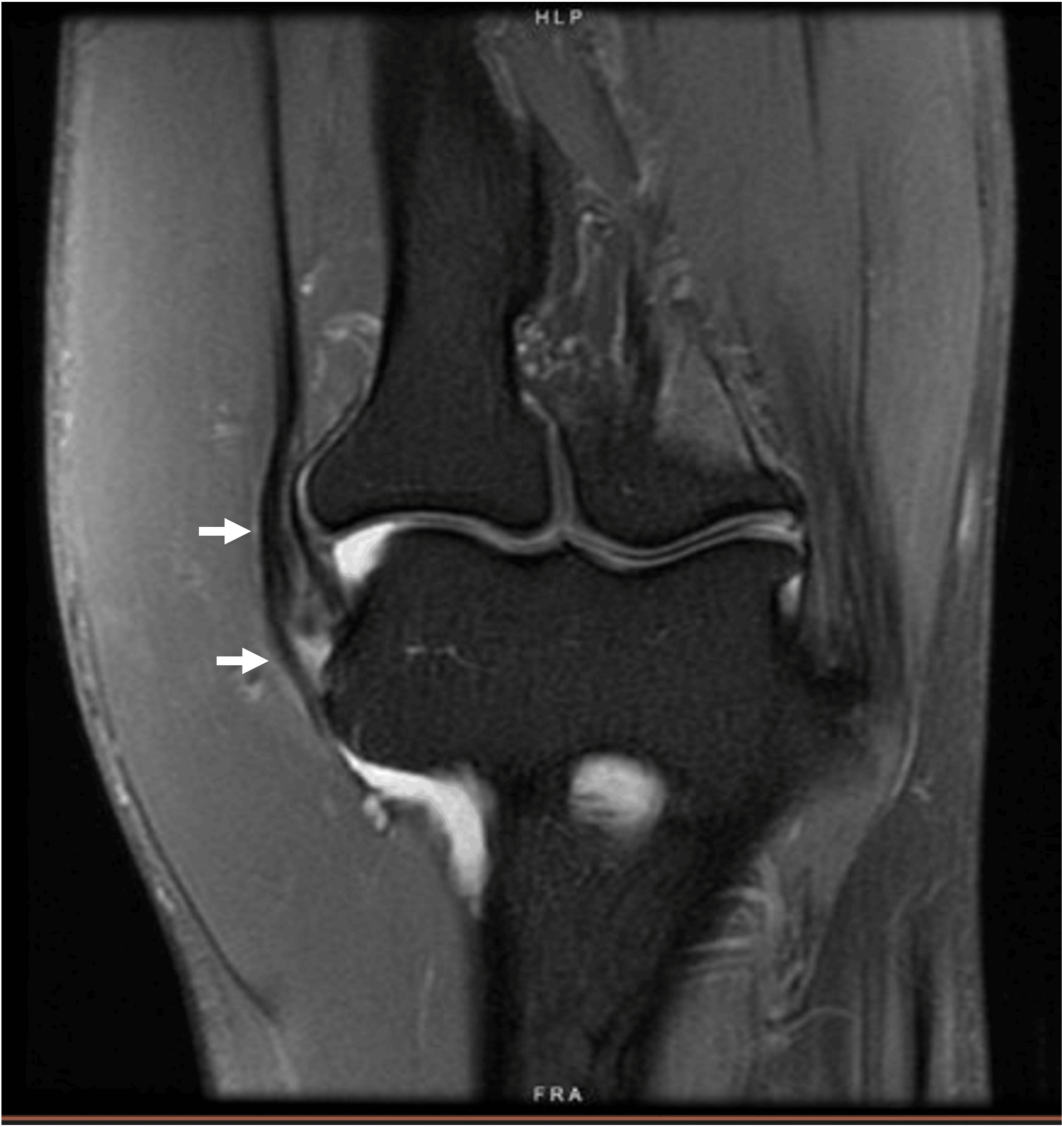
Tendinosis of the common extensor tendon at the lateral epicondyle attachment in a 53-year-old man. Coronal proton density fat-suppressed MRI of the elbow showing a low-grade partial thickness tear of the proximal common extensor tendon at the lateral epicondyle attachment. Arrows: Common extensor tendon.
